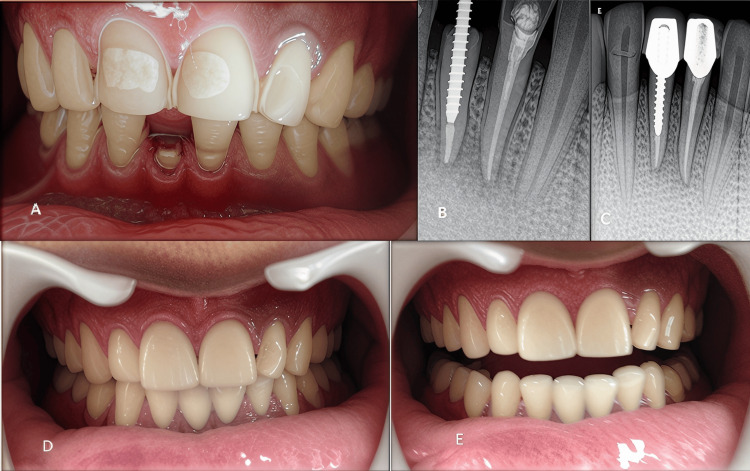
A) fractured tooth 41; B) radiographs for metal posts in 41 and RCT in 31; C) PFM crowns in mandibular teeth; D) postoperative photograph; E) Follow up after two years RCT: root canal treatment; PFM: porcelain fused to metal
